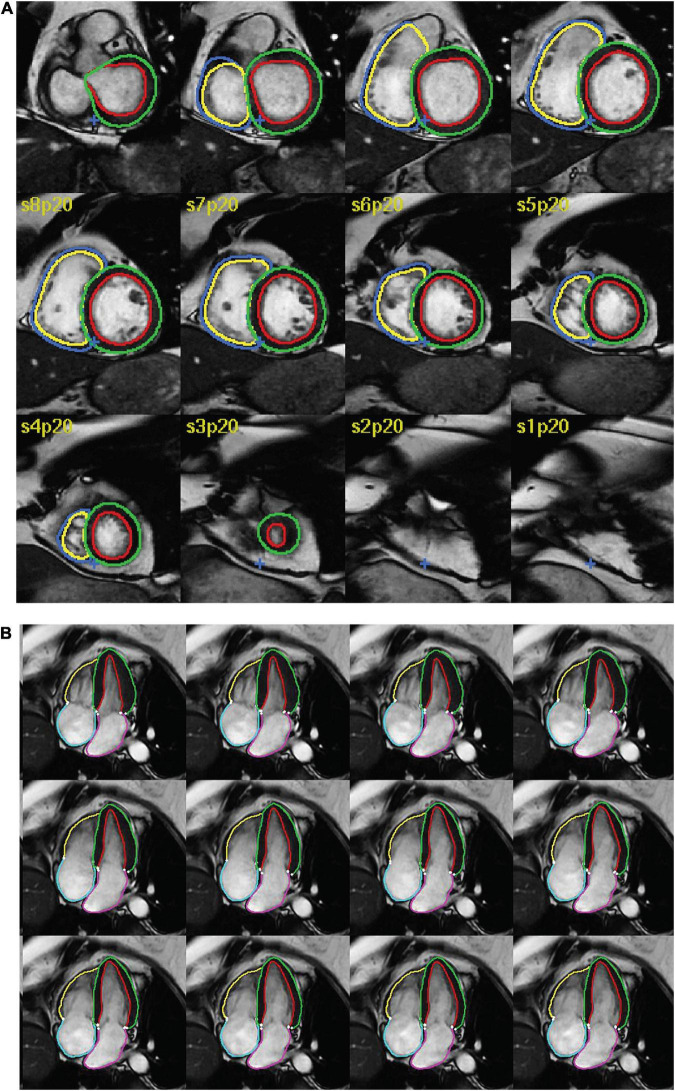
Examples of AI cardiac MRI segmentation. Examples of automatic (A) biventricular and (B) four-chamber segmentation. The colored contours in green and red show the left ventricular epi- and endocardium, respectively. The contours in dark blue and yellow show the right ventricular epi- and endo- cardium, respectively. The pink and turquoise contours outline the left and right atria, respectively.
